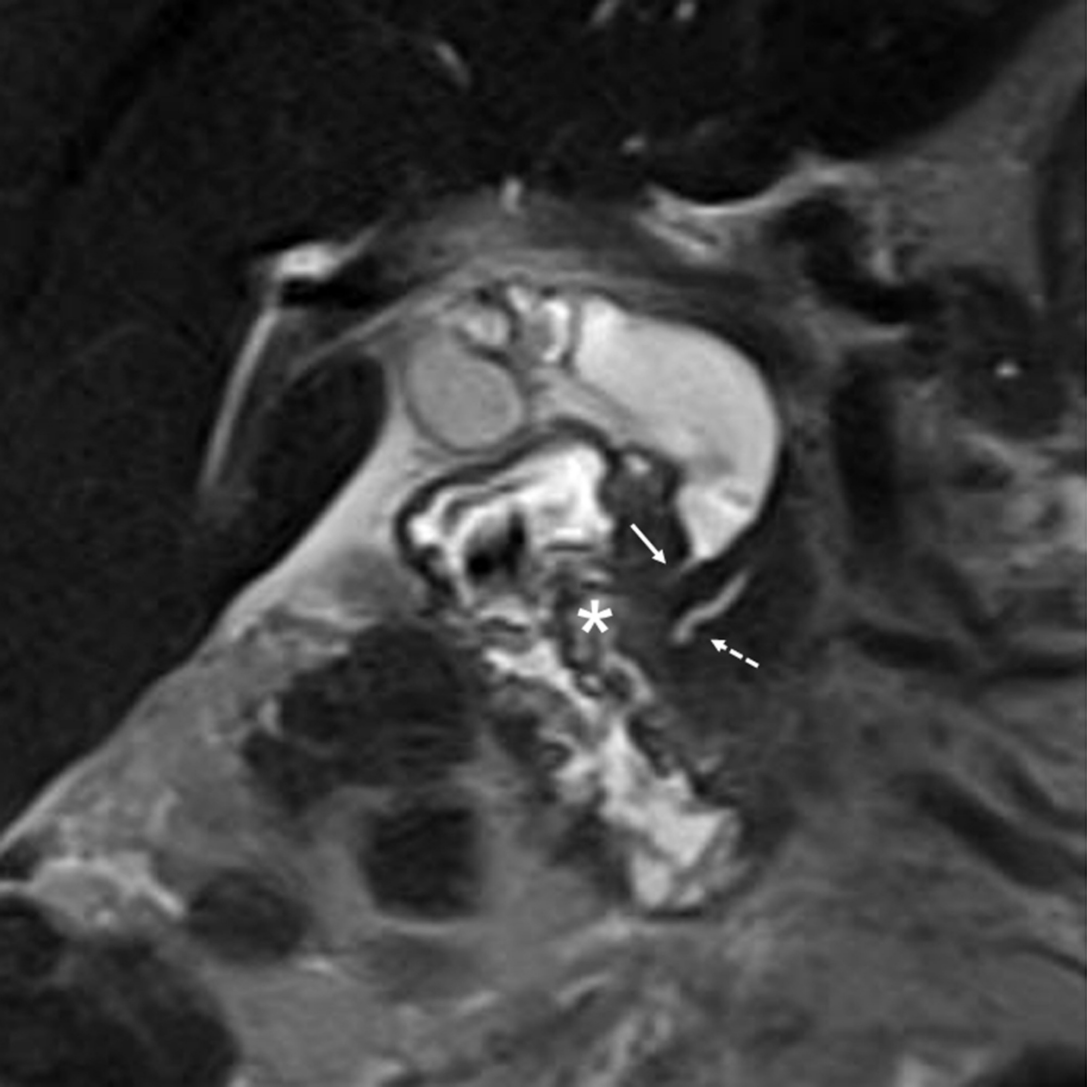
Magnetic resonance cholangiopancreatography (MRCP). The cystic duct and common bile duct are markedly distended with a smooth and short narrowing (solid arrow) at the distal aspect. No biliary stone is identified. The main pancreatic duct (dotted arrow) is not involved. Along the distal common duct, there is an area of ill-defined increased T2-weighted signal. The adjacent duodenal wall (*) shows prominently increased T2-weighted signal that reflects oedema which can be reactive to an infiltrative disease.
